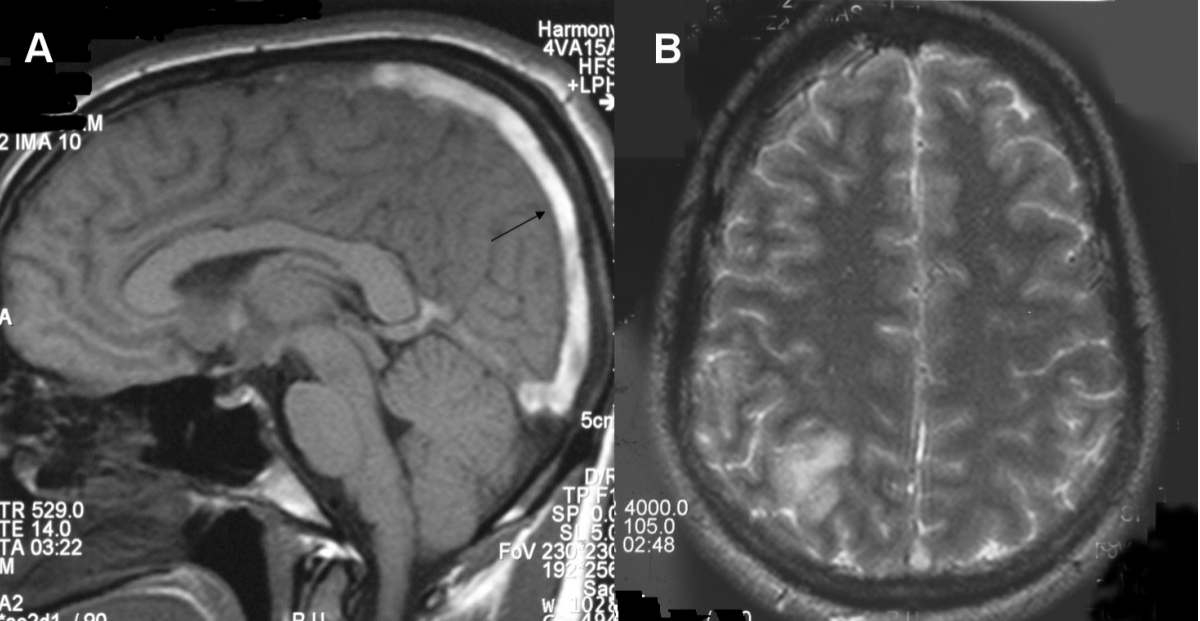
A. T1 weighted MRI - The superior sagittal sinus shows a hyper-intense signal of the thrombus seen in place of low-signal flowing blood. B. T2 weighted MRI showing venous infarct in right parieto-occipital region.
